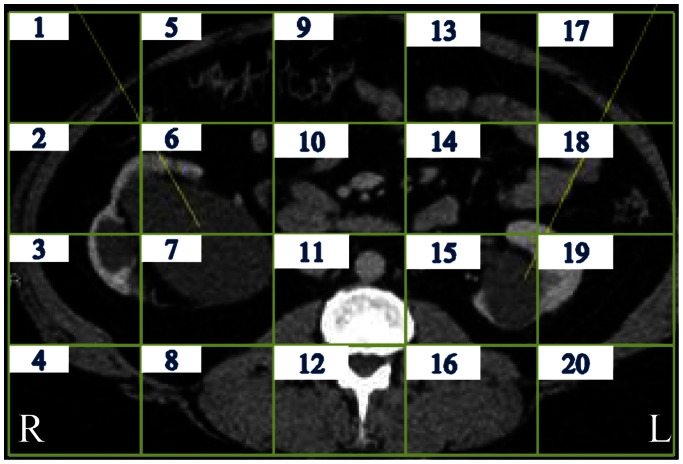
Cross-sectional abdominal CT-image depicting hydronephrosis. The hydronephrosis is present at the right side in area 2, 3, 6, and 7 and on the left side in area 15.
